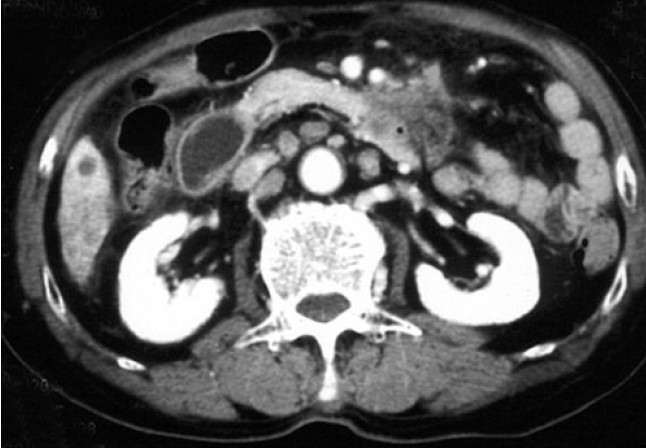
Computed tomography reveals a tumor in the third portion of the duodenum.
